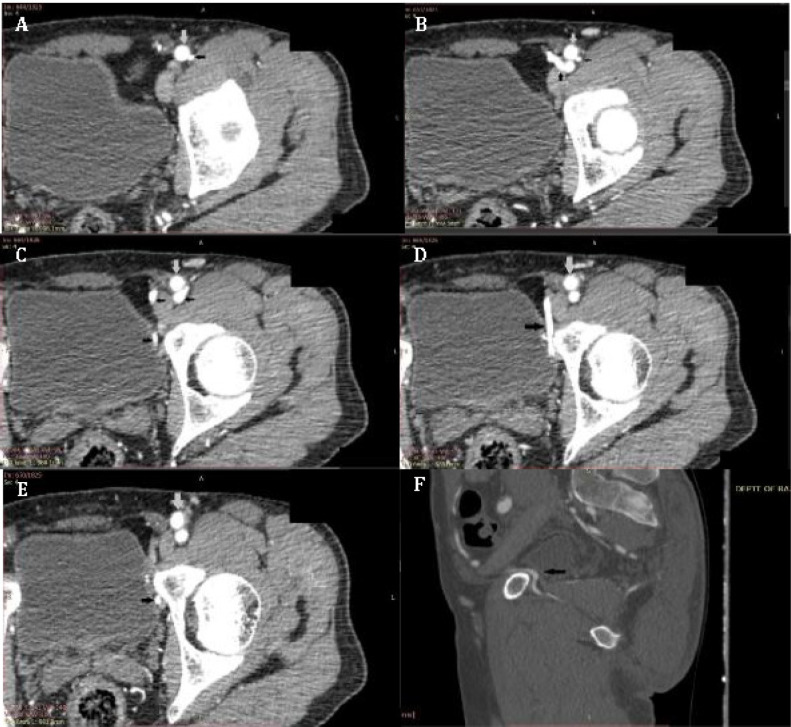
Sequential 2D axial sections (A-E; superior to inferior, soft tissue window) and (F) sagittal view (bone window) of CT angiographic images in a 35-year-oldman showing left Corona Mortis artery (black arrow; grey arrow depicts the external iliac artery).
